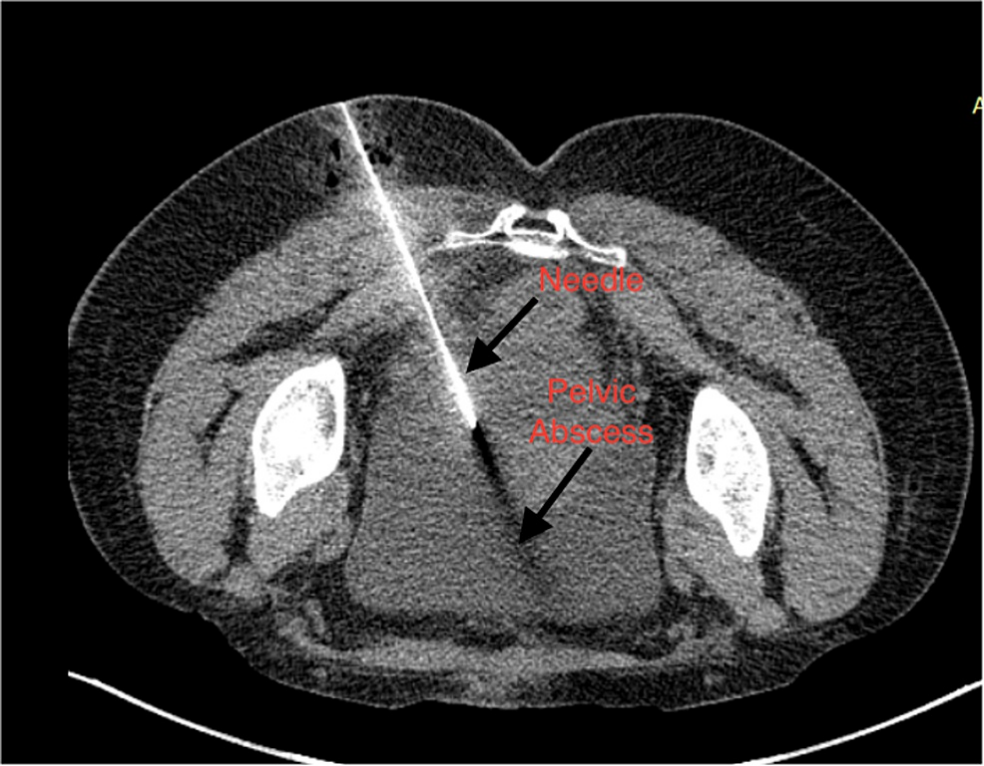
CT scan image shows initial needle placement in pelvic abscess via transgluteal approach.
